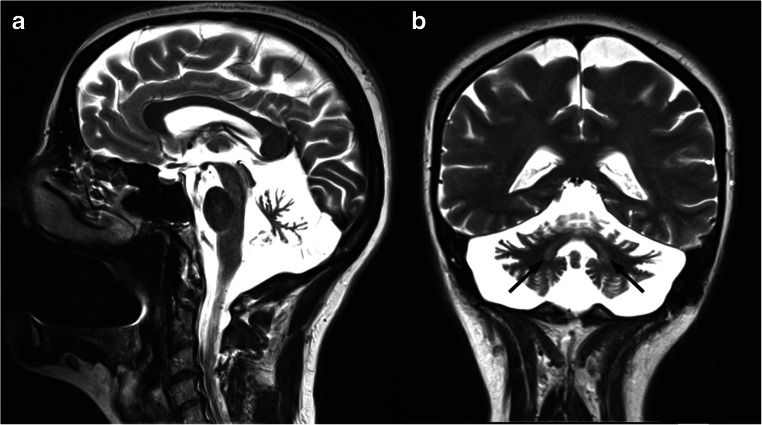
A 36-year-old female SCAR10 patient showing a global cerebellar atrophy, along with a mild T2-weighted hyperintensity affecting both dentate nuclei (black arrows in b)
